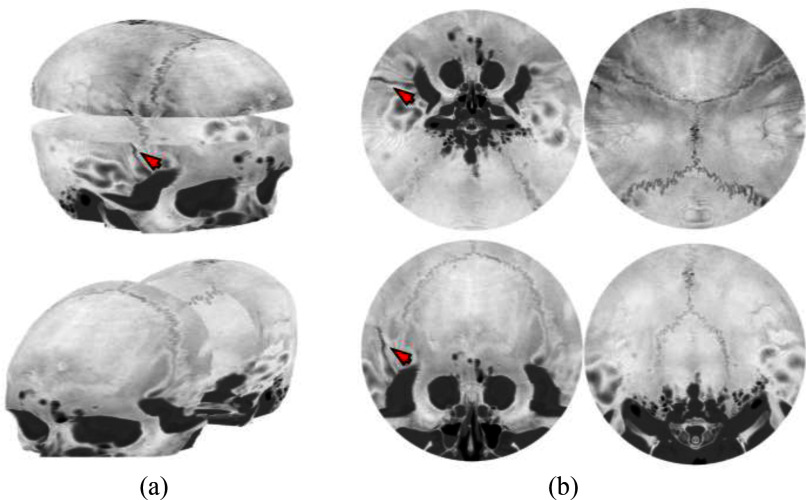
Skull mesh decomposition and flattening of trigonocephaly skull of Patient 134. (a) 3D skull mesh partitioned into LUFO regions (Lower, Upper, Front, Occipital) creating four simply connected open surfaces, each color-mapped with CT intensity values (HU). (b) Corresponding flattened representations obtained via disk harmonic mapping, preserving the geometric relationships of each surface in 2D planar space. Arrows indicate a fracture at the temporal bone. In this case only 1 out of 3 radiologists has correctly identified the fracture.
